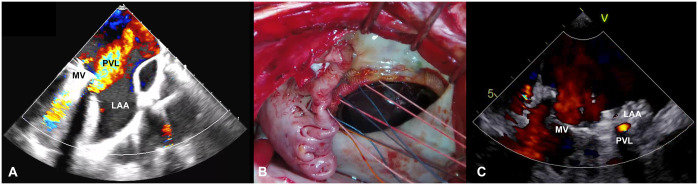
Figure1: (A) Preoperative echocardiogram. (B) An operative picture indicating the technique. (C) Postoperative echocardiogram. LAA: left atrial appendage; MV: mechanical valve; PVL: paravalvular leak.
